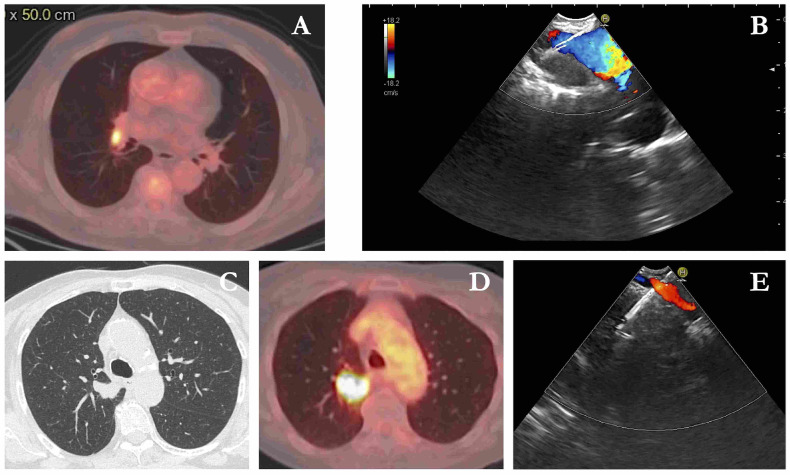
Positron emission tomography (PET)/computed tomography (CT) scan of a right interlobar lymph node (A) and transvascular EBUS−TBNA of the lymph adenopathy (B). CT (C) and PET/CT (D) scan of a right upper lobe pulmonary nodule and transvascular EUS−B-FNA of the lung lesions (E).
